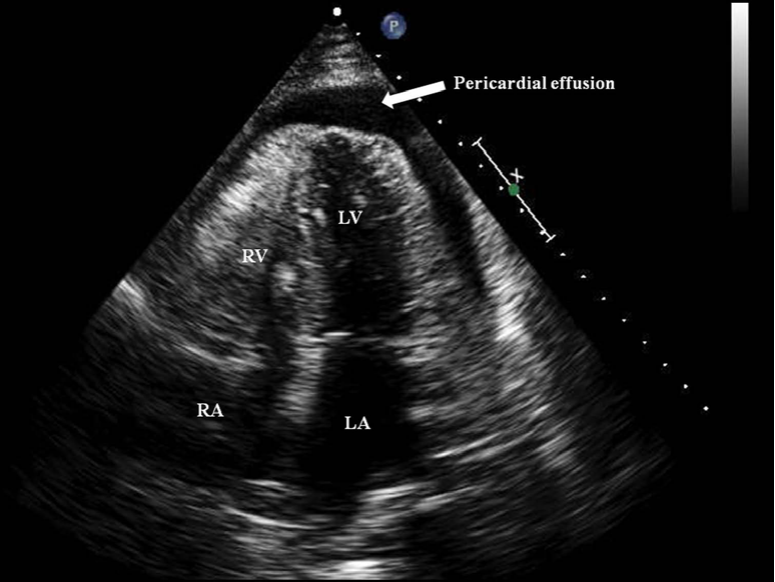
Transthoracic echocardiogram Apical 4 chamber views showing the pericardial effusion.
